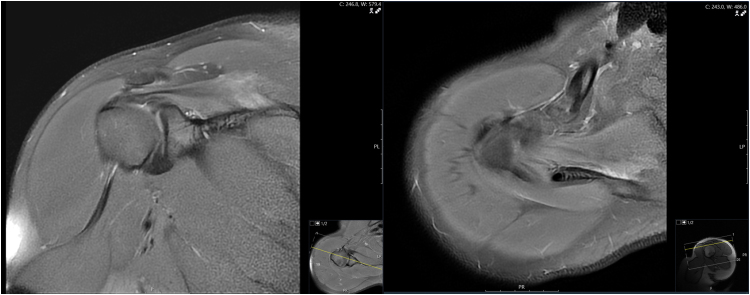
Magnetic resonance imaging of the right shoulder at 4 months post surgery showing reduced signal and size of supraspinatus. Coronal reconstruction (left) and axial (right) proton density (PD) weighted sequence.
